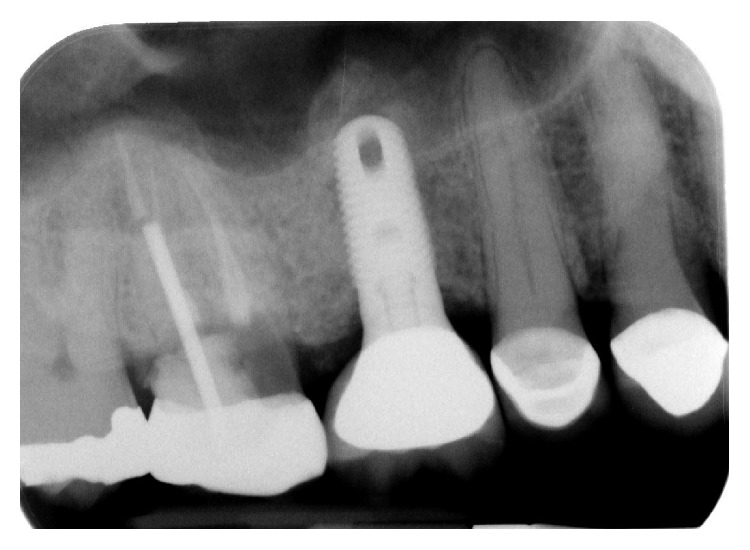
A periapical radiograph was taken after the implant placement. Bone condensation is noted apical to implant #3 due to the use of the osteotome sinus floor elevation technique. Mesial of #2, a deep unrestorable decay was observed.
